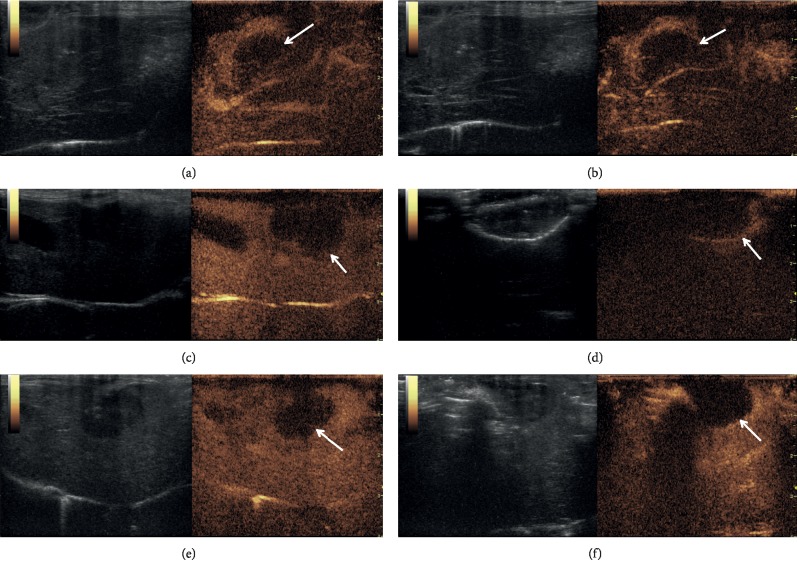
Contrast-enhanced ultrasound images of the tumors from three treatment groups before and after treatment. All liver tumors presented a nonuniform high enhancement model with weak enhancement at the centre (a, c, e) before treatment. After MEUS cavitation, tumor perfusion was decreased and the enlarged unenhanced perfusion defects appeared (b). After MWA ablation, a round-shaped enhancement defect lesion appeared (arrow in (d)). The liver tumor treated by using MEUS + MWA combination showed a much more regular and lower enhancement ablation zone (arrow in (f)).
